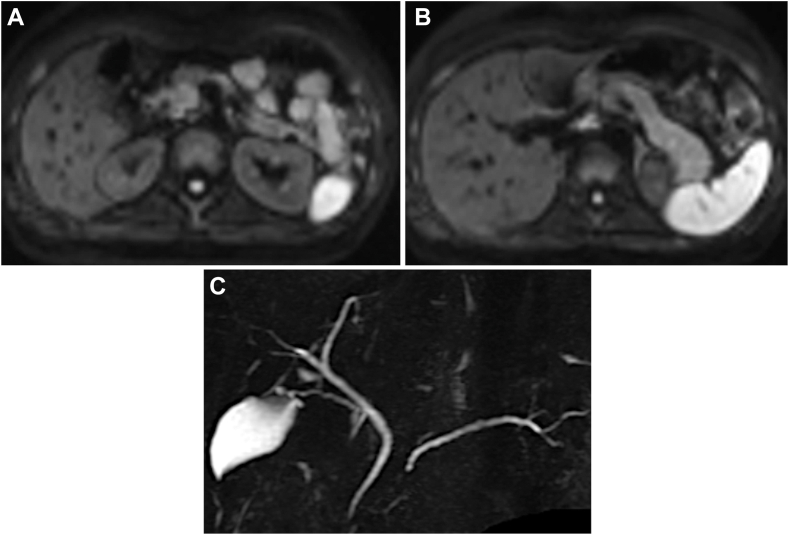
Magnetic resonance imaging images at the second admission. (A and B) DWI shows high-intensity areas in the pancreatic head (A) in addition to the body and tail (B). (C) Magnetic resonance cholangiopancreatography shows a narrowing of the MPD of the head and tail and slight enlargement of the body duct.
